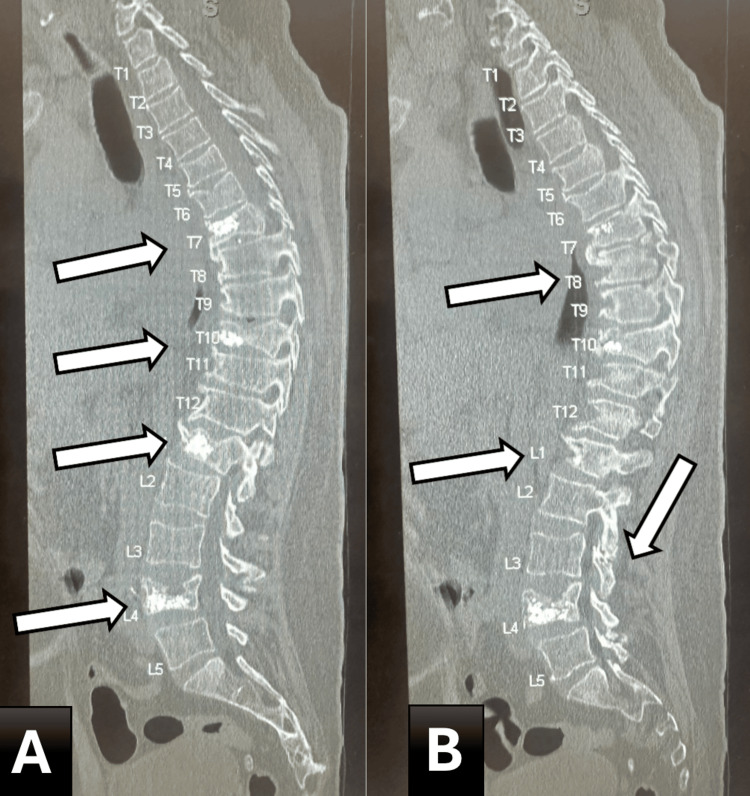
Multilevel vertebral compression fractures in the absence of focal osteolytic lesions. (A, B) Sagittal CT reconstructions of the thoracic and lumbar spine demonstrating multiple vertebral compression deformities (arrows), involving levels T7, T8–T11, and L1–L4. Several vertebral bodies demonstrate post-kyphoplasty changes with hyperdense cement material. Despite extensive structural compromise and multilevel involvement, no discrete osteolytic lesions are identified, further supporting an atypical radiographic presentation of multiple myeloma characterized by diffuse marrow infiltration rather than focal bone destruction.
